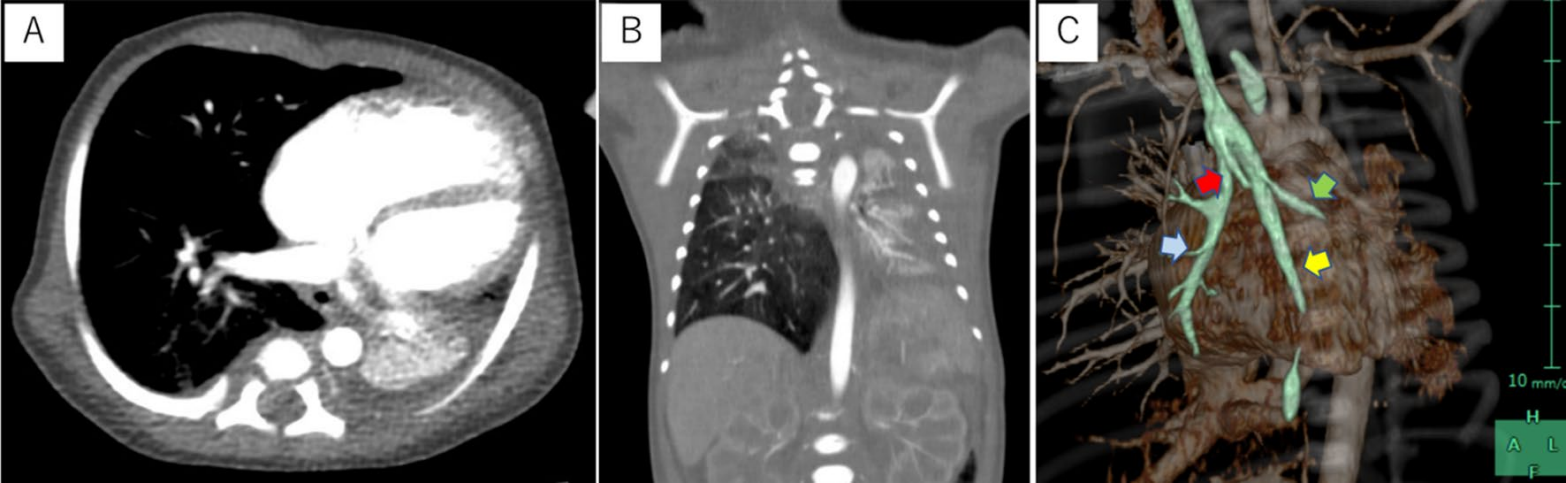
Contrast-enhanced computed tomography (CT) showing an atelectatic unilobe in the left lung (A, B). Three-dimensional CT image representing the tracheal trifurcation in three directions; the right main bronchus (blue arrow), blind-ended branch considered to be the left main bronchus (red arrow), and dorsal branch bifurcated to left lower lobe bronchus (green arrow) and the lower esophagus (yellow) (C)
